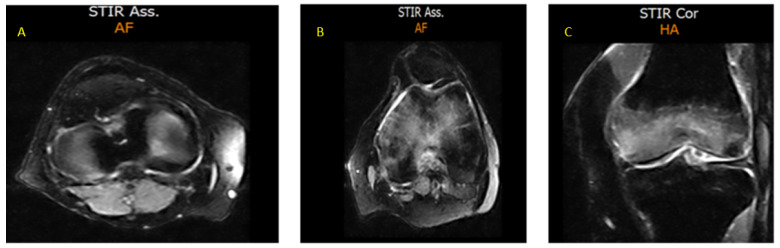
MRI sections of both knees, documenting BME (February 2022). (A) Axial STIR section of the right knee showing a hyperintense intra-spongious signal within the medial femoral condyle, consistent with localized BME; (B) Axial STIR section of the left knee demonstrating a more extensive hyperintense area of intra-spongious BME, affecting the medial femoral condyle and spreading towards the subchondral region, without associated cortical disruption; (C) Coronal STIR section of the left knee which further highlights the patchy, ill-defined margins of the BME, with a distribution typical of mechanical overload or subchondral stress reaction.
